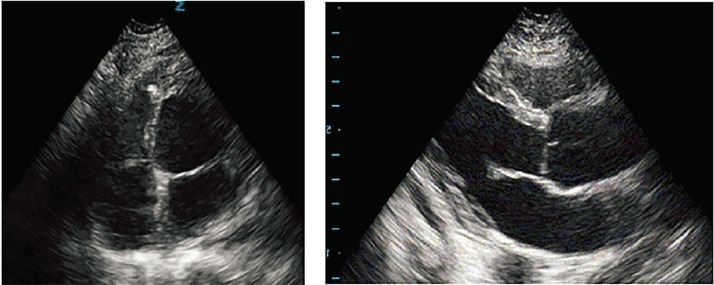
Point-of-care echocardiogram with an apical four-chamber view (left) and a parasternal long-axis view (right).
